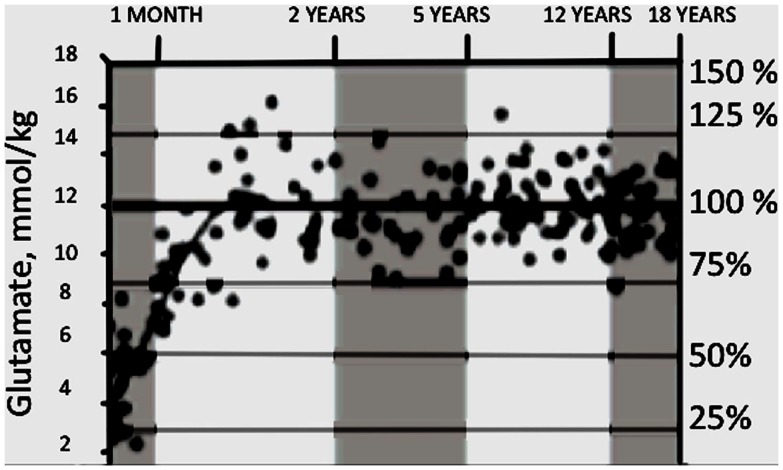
Developmental changes in glutamate content in parietal/occipital gray matter measured by MRI in young children. On percentage scale 100% refers to ages between 2 and 18 years. From Blüml et al. (2013).
